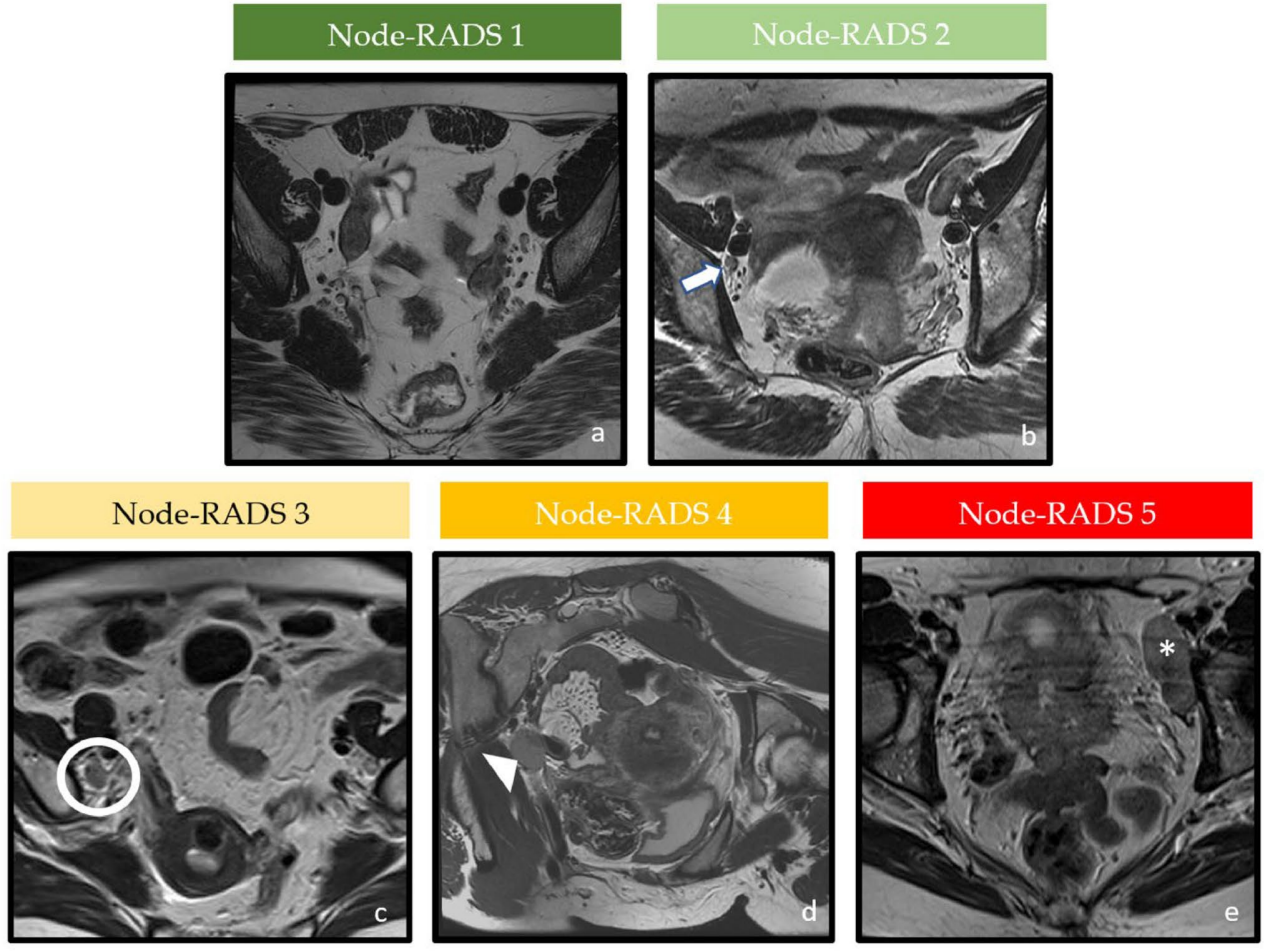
Axial T2-weighted images of pelvic lymph nodes with different Node-RADS score according to suspected malignancy. a Node-RADS 1: left external iliac LN with normal size and configuration. b Node-RADS 2: left external iliac LN (arrow), showing a short axis of 5 mm and homogeneous signal intensity. c Node-RADS 3: the left external iliac LN (circle), showing a short axis of 8 mm, heterogeneous signal intensity with oval shape without fatty hilum. d Node-RADS 4: left external iliac LN (arrow), showing a short axis of 13 mm with heterogeneous signal intensity and spherical shape without fatty hilum. e Node-RADS 5: right obturator bulky LN (asterisk) with long axis > 30 mm, corresponding to a high risk of malignancy.
